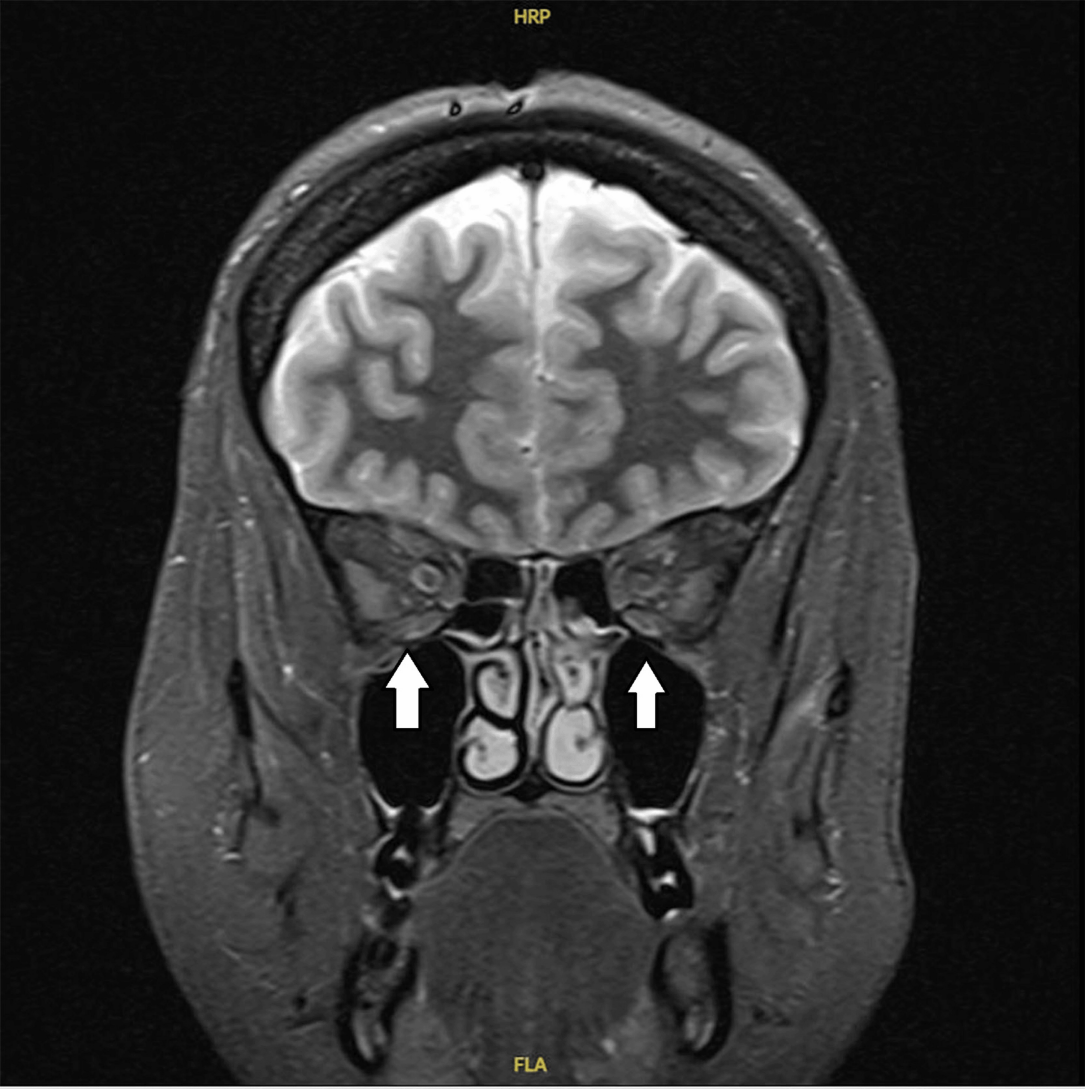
Coronal T2-weighted MRI image demonstrating bilateral optic nerve sheath prominence. The optic nerve sheaths appear distended, a radiological sign often associated with raised intracranial pressure. MRI: magnetic resonance imaging
